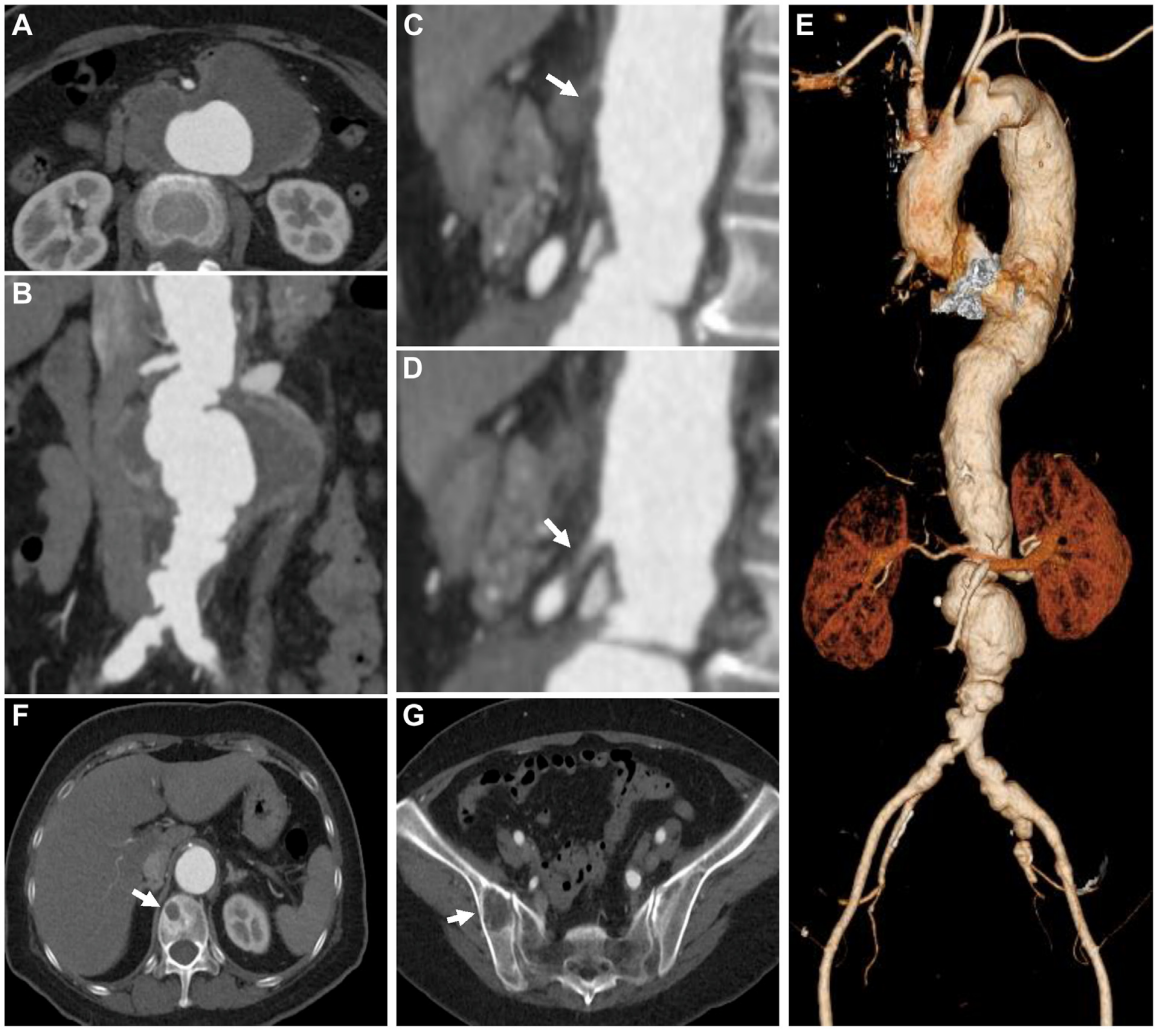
Preoperative computed tomography (CT). (A) Irregular and lobulated para-renal abdominal aortic aneurysm (PRAAA) with irregular and multilobulated shape, without periaortic soft-tissue density and inflammation surrounding the aneurysm on the axial image. (B) PRAAA on the coronal image. (C) Occluded celiac artery (CA) (*arrow*) on the sagittal image. (D) Stenotic superior mesenteric artery (SMA) (*arrow*) on the sagittal image. (E) Three-dimensional reconstruction of the aorta. (F) Osteolytic changes in the 12th thoracic vertebra (*arrow*) on the axial image. (G) Mass in the iliac bone (*arrow*) on the axial image.
